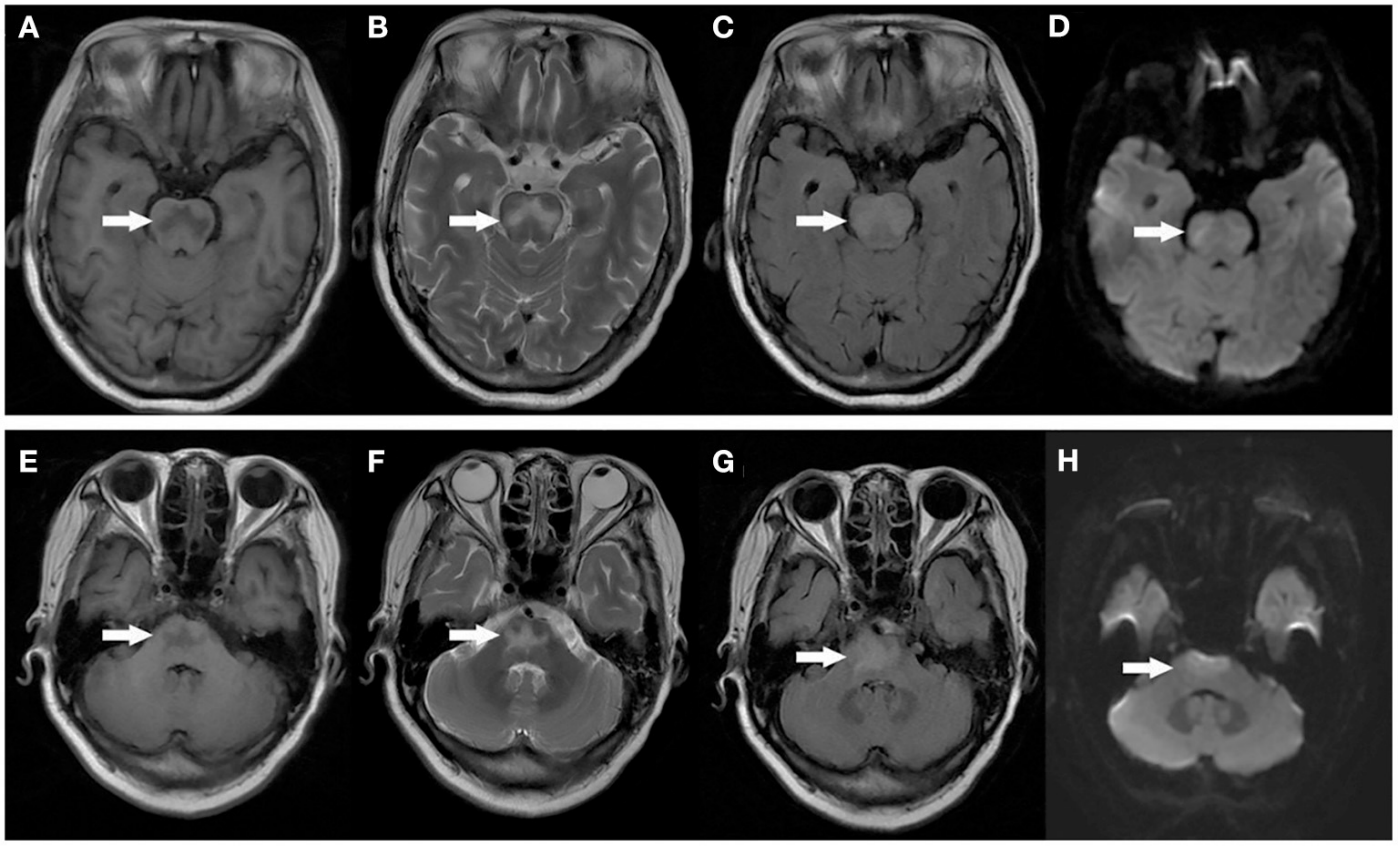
MRI image of the patient's head: Axial T1 weighted images demonstrating symmetric low signal in the central pons (A,E). Axial T2 weighted images demonstrating symmetric high signal in the central pons (B,F). T2 FLAIR images demonstrate symmetric high signal in the central pons (C,G). DWI images didn't show any diffusion restriction in the central pons (D,H).
